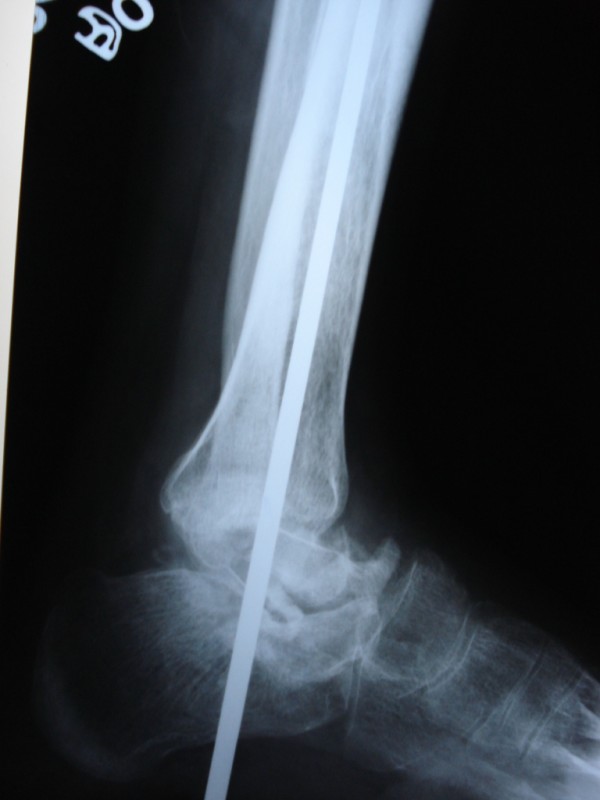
Immediate postoperative radiograph showing talectomy, bone grafting and stabilization with a Steinmann pin.
